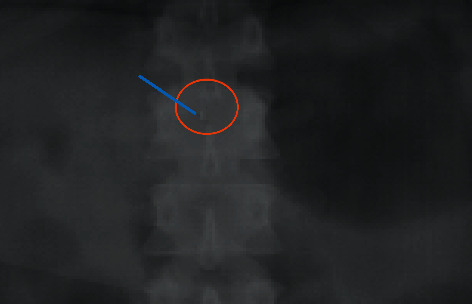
AP lumbar spine radiograph.
